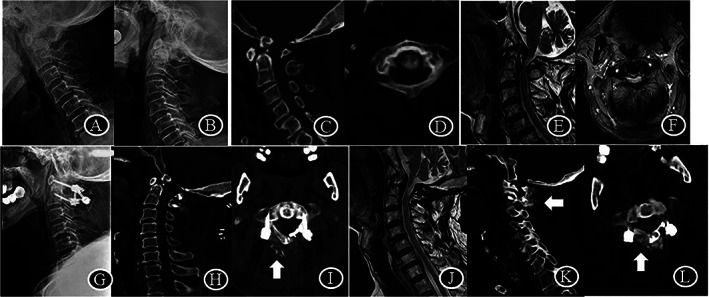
Radiological data of patient no. 1 with atlas hypoplasia and os odontoideum. (A–D), ISD of 23 mm, SAC of 3 mm and ADI of 4 mm pre‐operation; (E, F), T2–weighted images show constriction of the dural sac at the level of the atlas; (G–I). ISD of 30 mm, SAC of 20 mm and ADI of 1 mm immediately post‐operation. Note the autogenous iliac bone graft; (J). 24 months later after surgery, MRI shows subarachnoid space around the dural sac at the level of the atlas; (K, L). The reduction and decompression maintained at the last follow‐up. The healing of the hinge fracture and the fusion between C1 posterior arch and C2 lamina was identified
